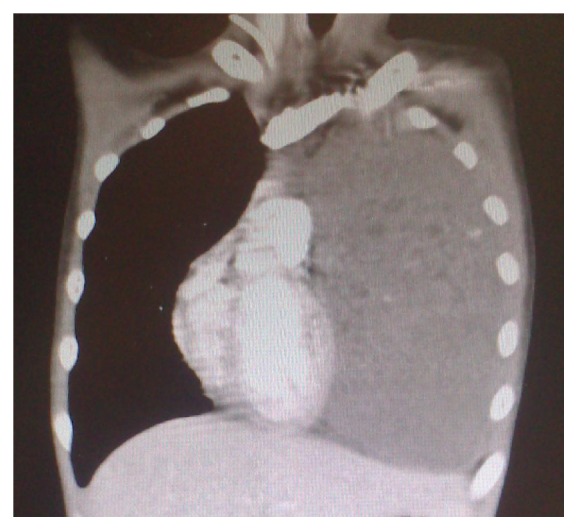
Coronal view of the CT scan showing that the mass occupies the whole of the left thoracic space with mediastinal shift to the right.
